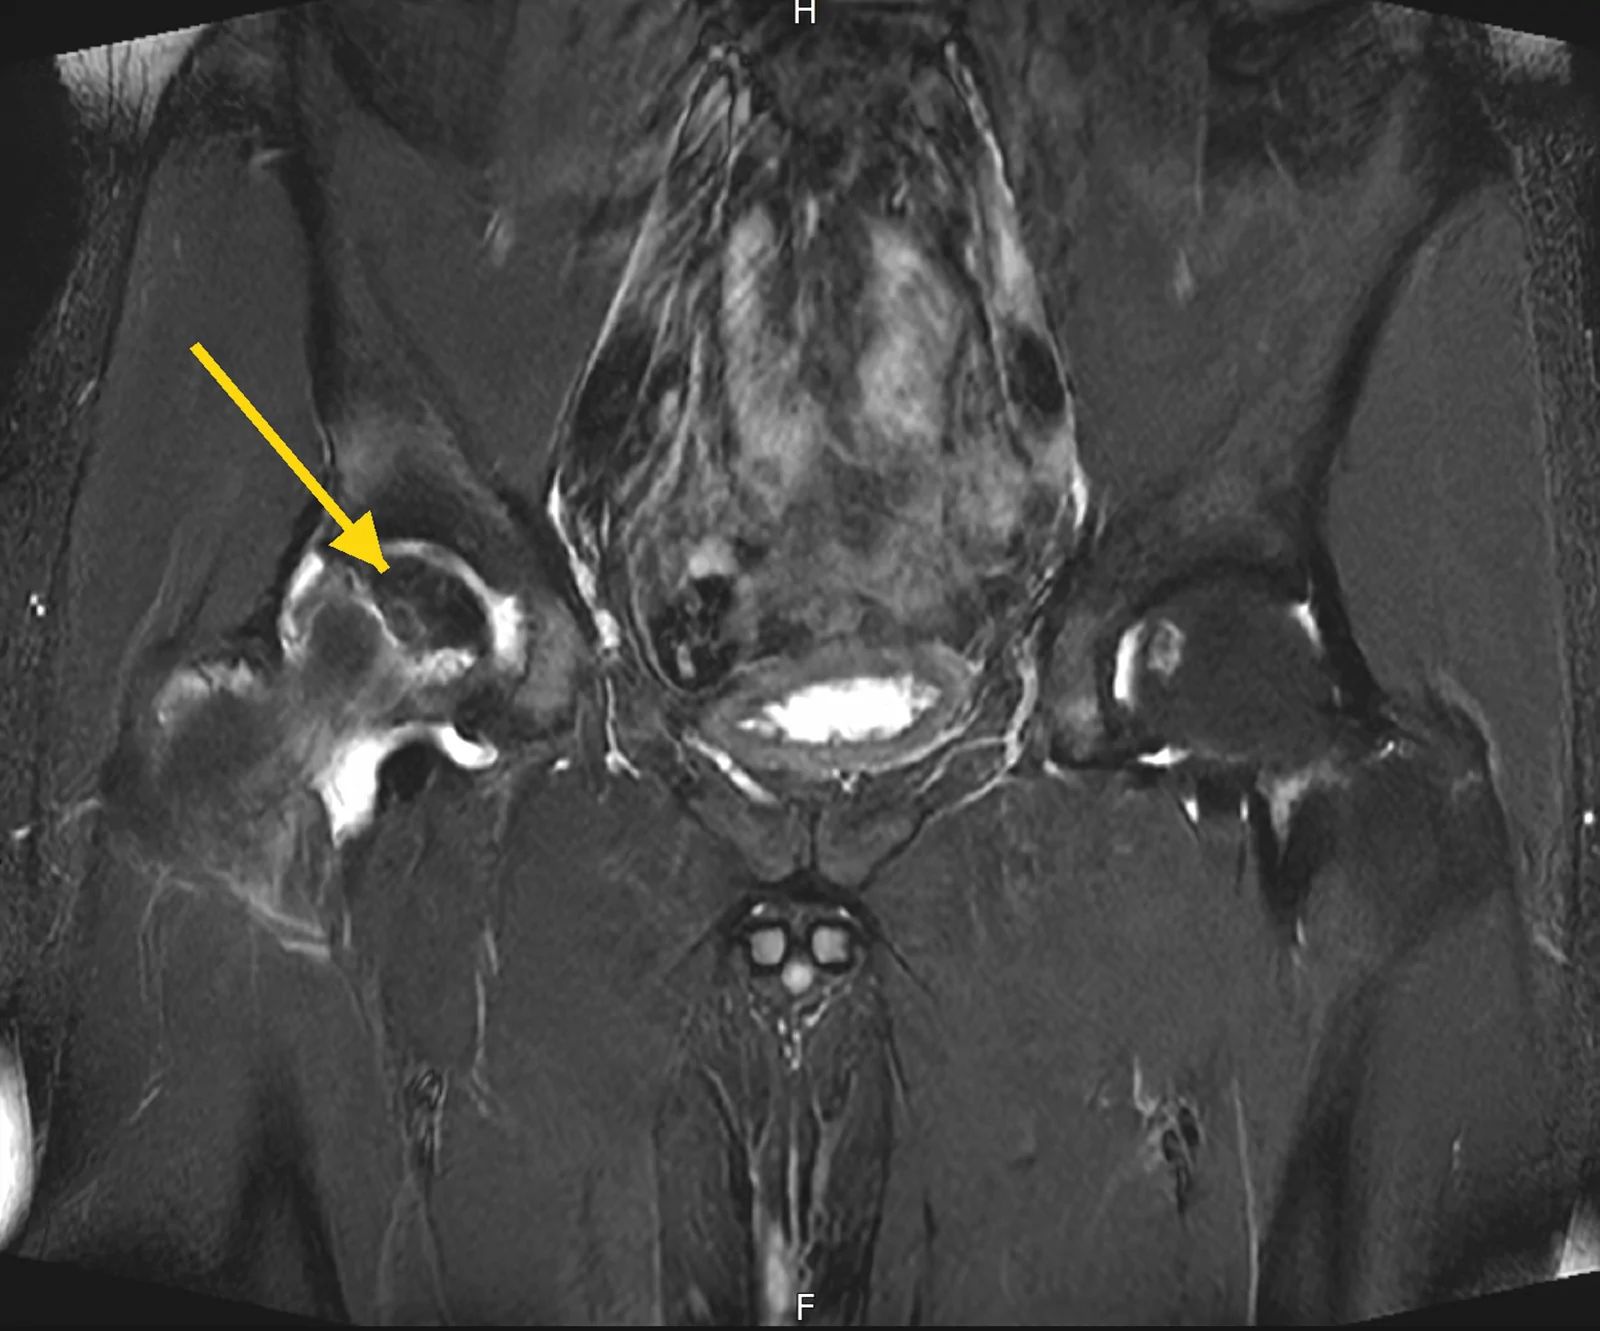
Coronal fat-suppressed MR image The arrow indicates the right femoral head with bone marrow edema; a large joint effusion and synovitis are present in the right hip.
